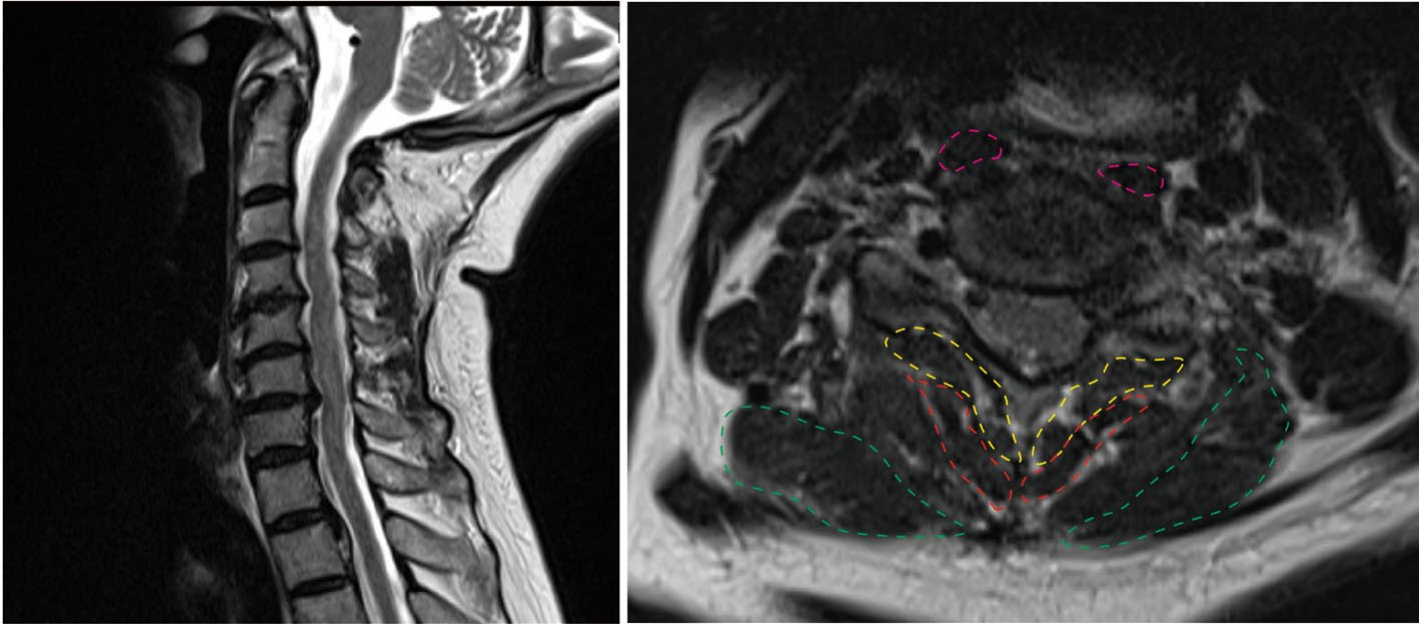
Magnetic resonance images depicting cervical spondylotic myelopathy. Left and right images show the transverse relaxation time (T2)‐weighted sagittal, and axial images of the cervical spine (fifth/sixth cervical vertebral [C5/6] disc level), respectively. Magenta, red, green, and yellow, depict the longus colli, semispinalis cervicis, splenius capitis, and multifidus muscles, respectively.
